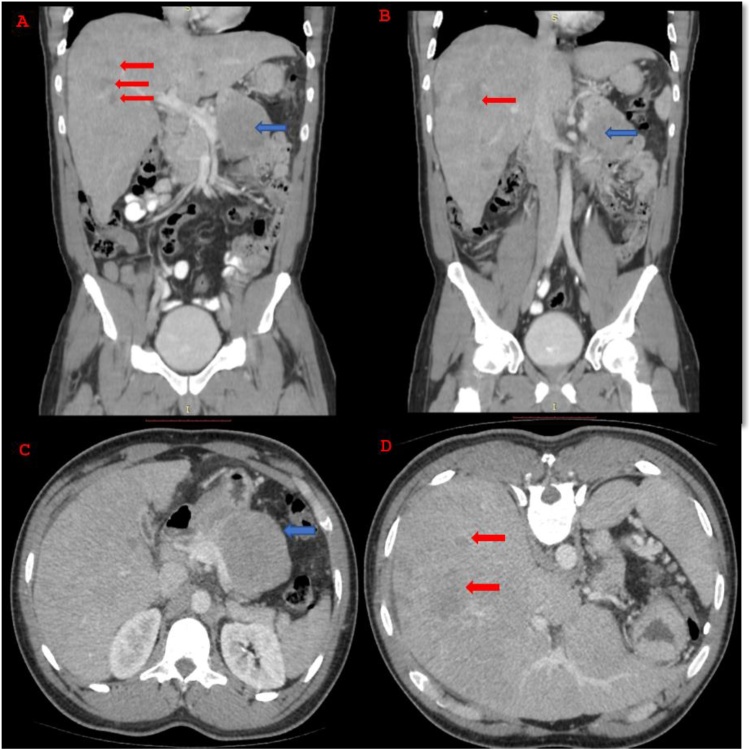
Abdominal CT Images. A and B: Coronal images: Hepatomegaly with vague rim-enhancement of the hepatic lesions (red arrows). The complex cystic lesion in the pancreas (blue arrow). No suspicious metastatic bony lesions were visualised. C and D: Axial plane (contrast): C, well-defined hypodense lesion (blue arrow) in the pancreatic body; D, poorly circumscribed hypodense liver lesions of variable sizes throughout the liver parenchyma (red arrow) that do not follow any particular enhancement pattern. There is no dilatation of the biliary ducts.
